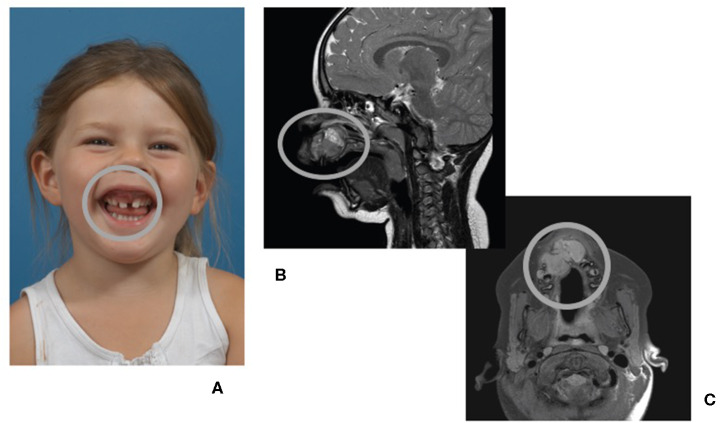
A 4-year-old child presenting with a fast growing tumor (circled in all panels) of the anterior maxilla. (A) Front view of patient, smiling. (B) Magnetic resonance imaging (MRI) of the head, midsagittal plane. (C) MRI of the head, coronal plane.
